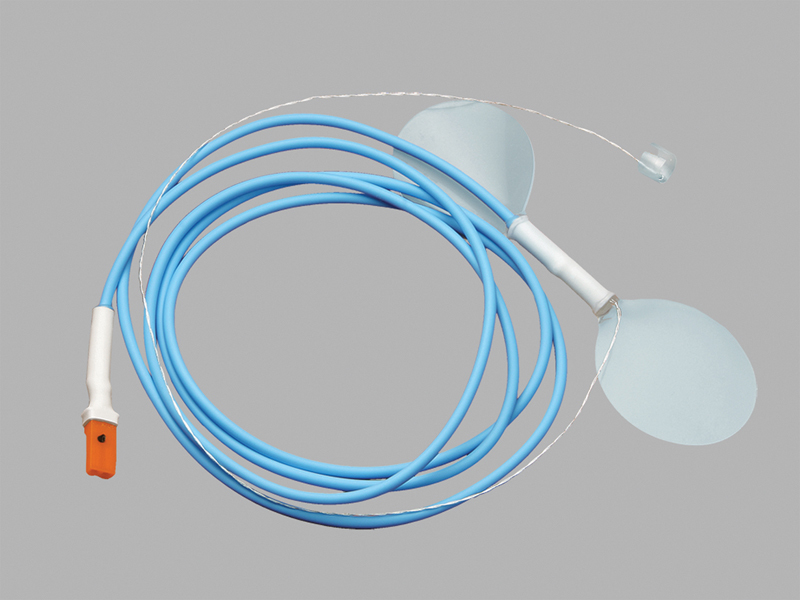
Cook–Swartz Doppler probe.
